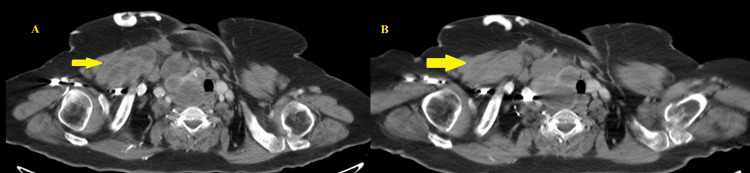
Comparison of CT imaging from May 2025 (A) to August 2025 (B) showing the interval resolution of cystic component in the right pectoralis major muscle CT: computed tomography
